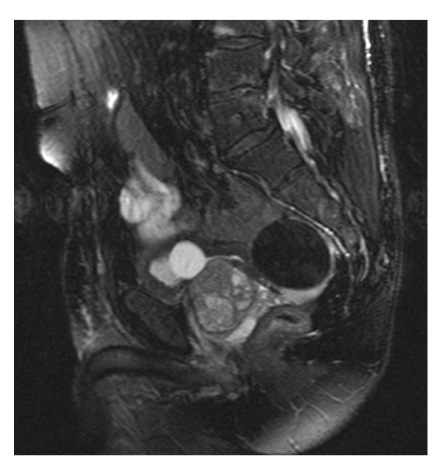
T2-weighted (fat-suppressed) sagittal image of the pelvis reveals a 5.5 cm retrovesical lesion lying anterior to the rectum. It is well circumscribed. There is no pelvic sidewall lymphadenopathy.
